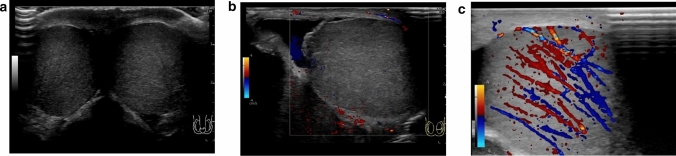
Ultrasonographic images of a 13-year-old boy with left testicular torsion. a The left testis has an abnormal axis. b Absence of blood flow within the testis is evident. c Outward manual detorsion by 720° is performed. Hypervascular flow within the whole affected testis is evident throughout the testis. Movie before manual detorsion; ultrasonogram shows outward twisting of the left spermatic cord (curved arrow). The spermatic cord shows 720° twisting. Manual detorsion was performed in the inward direction based on these findings
